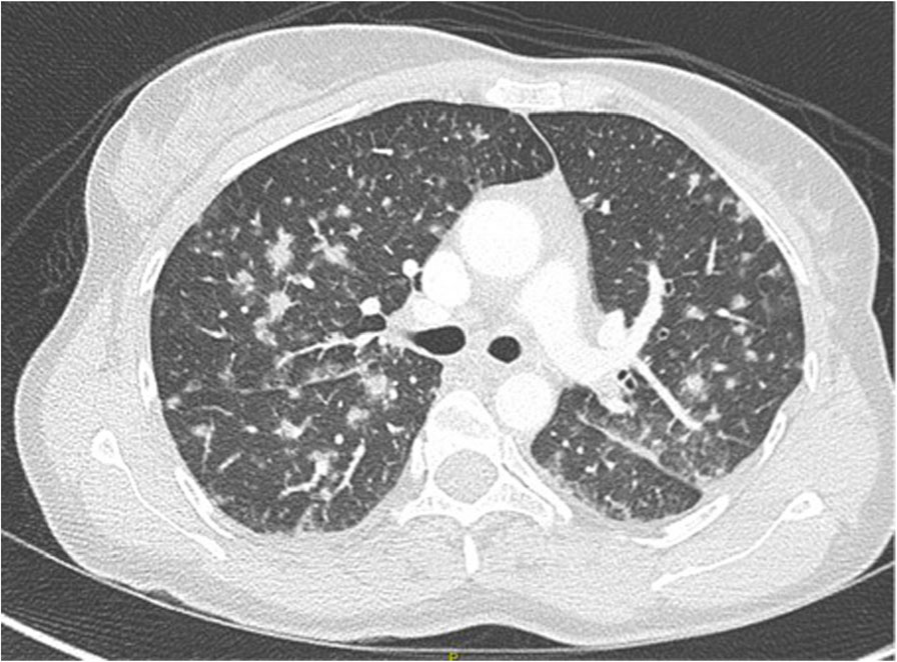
Imaging characteristics from lung CT. A 46-year-old woman was admitted to the ICU for acute respiratory failure. She underwent kidney transplantation 12 years ago. She reported fever and a typical chickenpox skin rash 5 days before admission. The onset of respiratory symptoms started 2 days before ICU admission and invasive mechanical ventilation was implemented at day 1. She developed a severe ARDS requiring prone positioning, neuromuscular blockers, and 14 days of invasive mechanical ventilation. Lung CT scan demonstrated diffuse bilateral nodules, patchy ground glass opacities, and interlobular septal thickening. A fiber bronchoscopy with bronchoalveolar lavage documented a *Staphylococcus aureus* co-infection. She received intravenous aciclovir 10 mg/kg/8 h during 15 days associated with 10 days of oxacilline and was discharge alive from the ICU 17 days after admission
